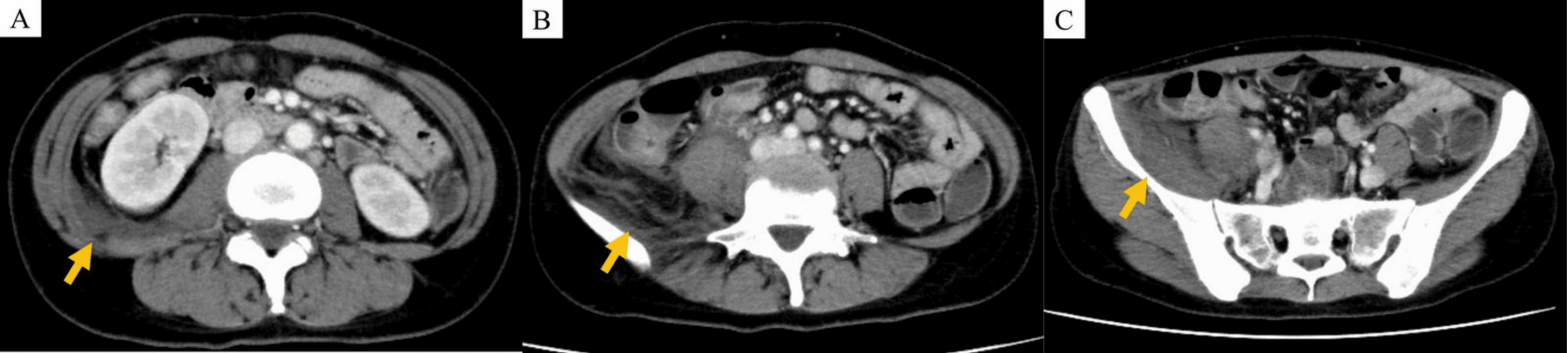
Contrast-enhanced CT findings at the initial visit (A-C) Fluid accumulation and increased fat density in the right retroperitoneal cavity.
